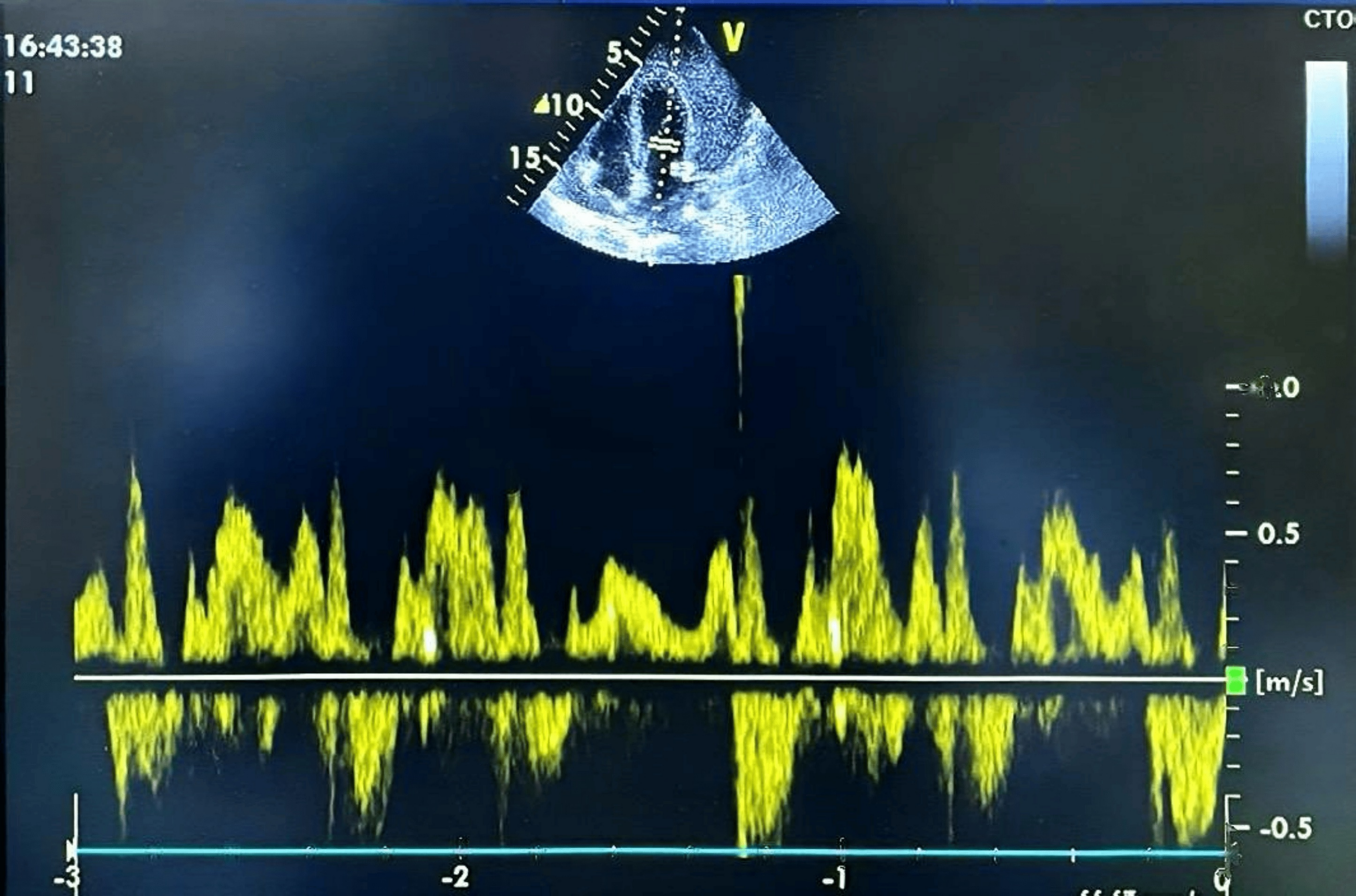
Mitral inflow variation. Pulsed-wave Doppler of the mitral inflow showing >50% respiratory variation. Combined with echocardiographic chamber collapse, these Doppler changes confirm significant respiratory dependence and support the diagnosis of cardiac tamponade.
